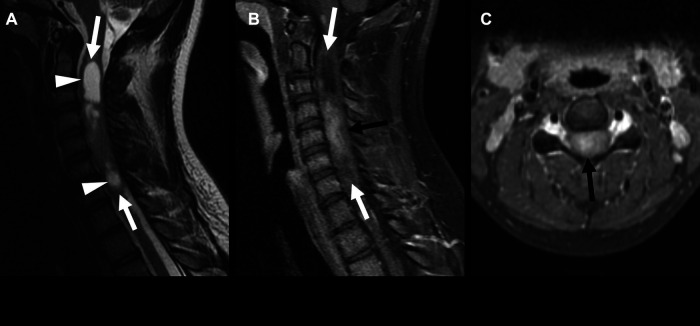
Spinal ependymoma, not elsewhere classified, in a 17-year-old girl with a history of neurofibromatosis 2, in the cervical spinal cord (arrows). Sagittal T2-weighted images show cystic areas (A) (arrowheads), and sagittal and axial postcontrast images show enhancement of the solid components of the tumor (B, C) (black arrows). There is an associated expansion of the spinal cord and effacement of the extra-axial spaces of the mid to distal cervical spine.
